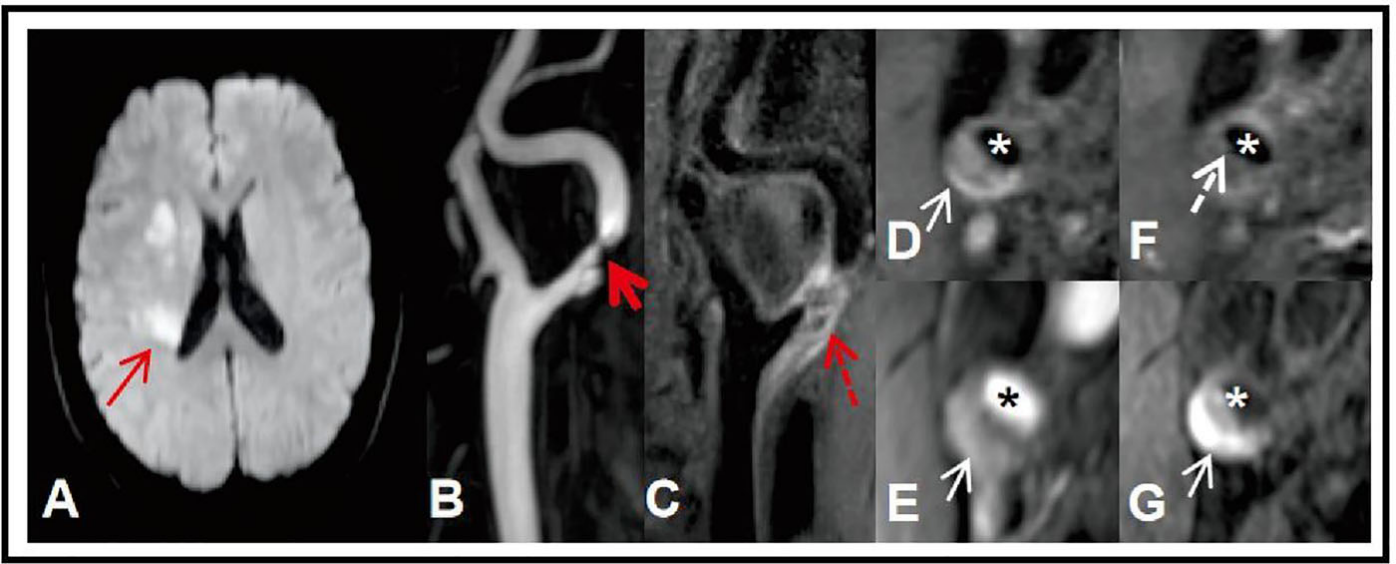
A representative case with stroke recurrence. A 59‐year‐old man with an ischemic stroke. The Serum Hs‐CRP level was 10.4 mg/L. He had an ipsilateral recurrence 10 months after discharge. DWI (A) showed a patchy hyperintensity lesion at the right corona radiata area (thin red arrow). CE‐MRA (B) showed severe stenosis at the right ICA (thick red arrow). Pre‐contrast T1W reformat image (C) displayed a large plaque involving the carotid bifurcation and the beginning of the ICA (red dotted arrow). NWI was 0.94. The plaque showed heterogeneous signal intensity, with a large area of hyperintensity on Pre‐contrast axial T1W (D), 3D TOF MRA (E), and 3D MP‐RAGE (G), indicating IPH (white arrow). Post‐contrast T1W (F) images showed a large non‐contrast area, suggesting LRNC with a thin/ruptured FC (white dotted arrow). 3D MP‐RAGE, 3D magnetization‐prepared rapid acquisition gradient‐echo; 3D TOF, 3D time‐of‐flight; CE‐MRA, Contrast‐enhanced magnetic resonance angiography; DWI, diffusion‐weighted imaging; FC, fibrous Cap; Hs‐CRP, high‐sensitivity C‐reactive protein; ICA, internal carotid artery; IPH, intraplaque hemorrhage; T1W, T1‐weighted.
